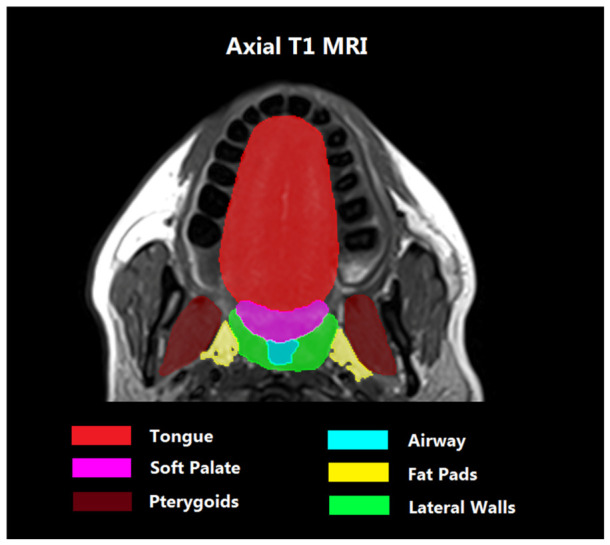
Soft Tissue Lengths. Mid-sagittal T1 MRI demonstrating measurement of tongue and soft palate length. Tongue length was acquired along the major axis, defined as the span between the most anterior and posterior points of the structure. Soft palate length was acquired along the curvilinear distance between the posterior nasal spine and distal end of uvula.
